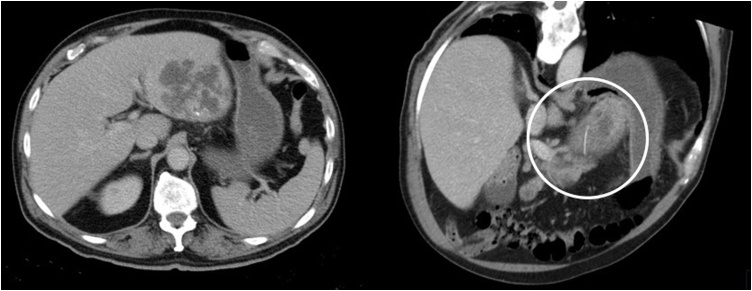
Abdominal CT scan at admission. A multiloculated hepatic abscess in the left lobe is noted with a high-density linear image suspected of being a foreign body.
